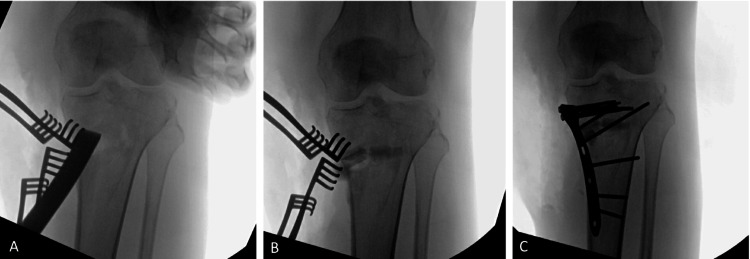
Intraoperative X-ray series showing a medial opening wedge proximal tibial osteotomy. Image A shows the elevation of the proximal tibia. Image B demonstrates the placement of the fibula strut. Image C shows the final position of the plate and screws.
